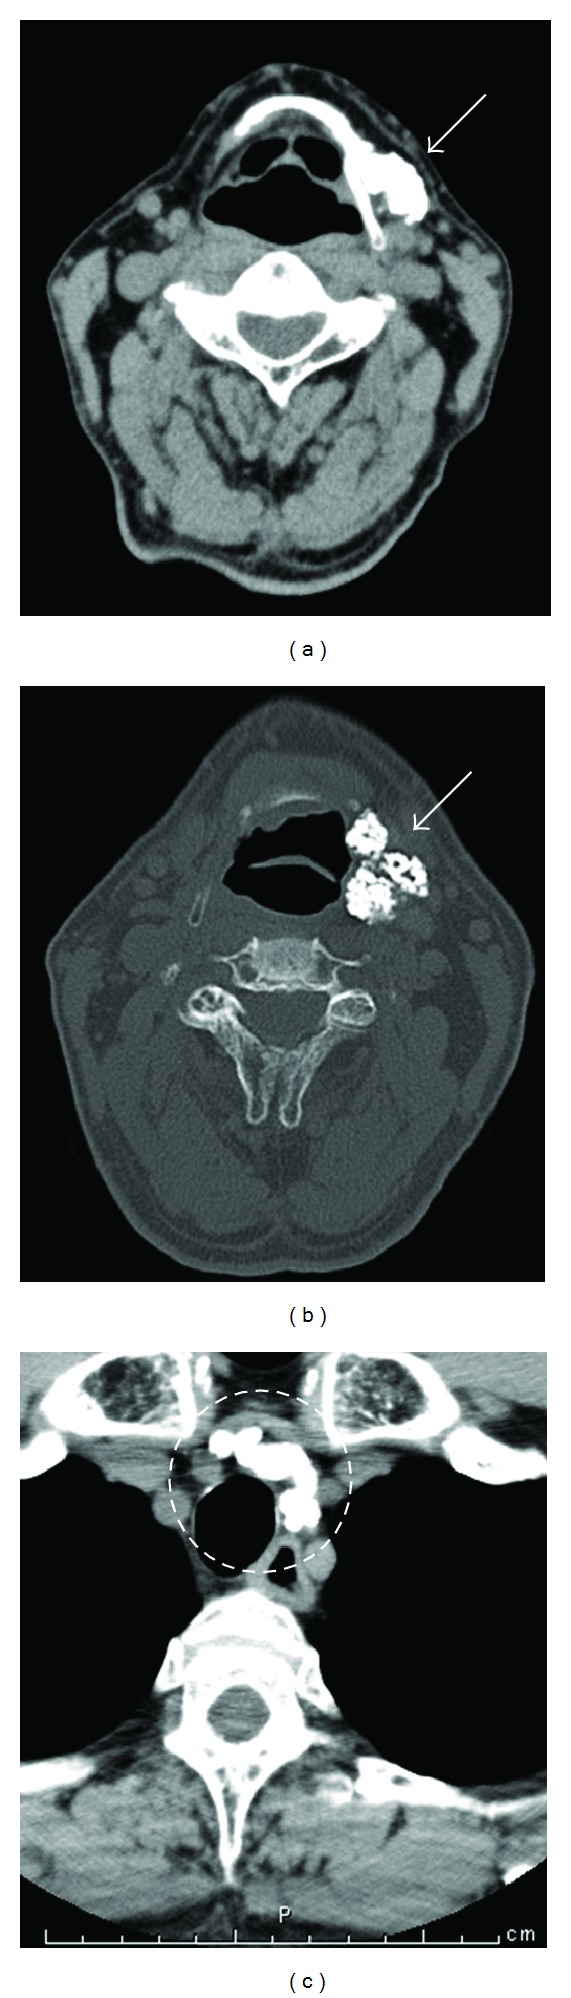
Neck and chest CT. Nodular shadows with calcification were observed (arrows in (a) and (b) and circles in (c)). ((a), (b)) Submandibular region. (c) Upper mediastinum.
